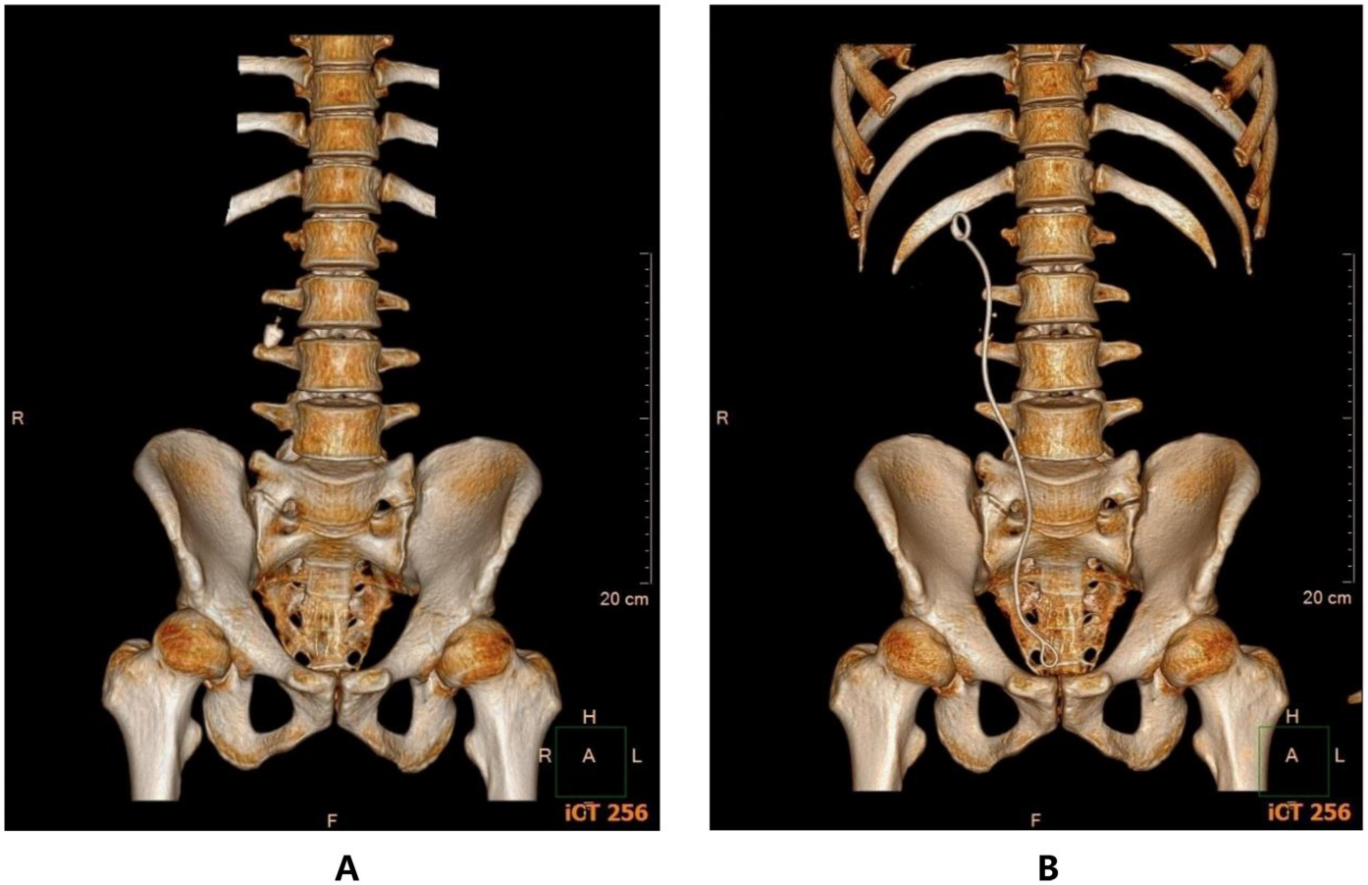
Comparison of patients' CT 3D reconstructed images: (A) pre-operation. (B) post-operation.
